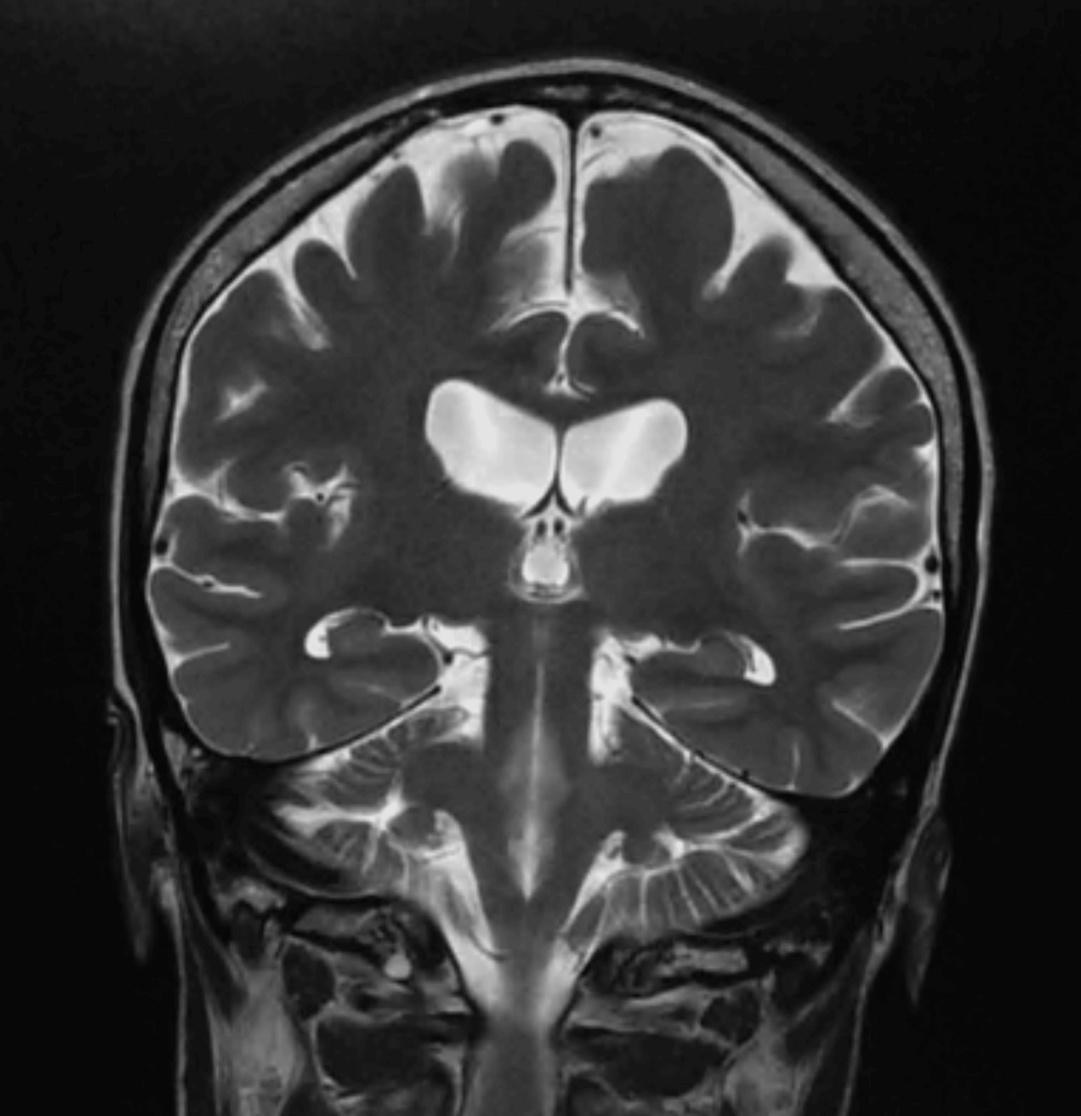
MRI brain shows normal hippocampus.
